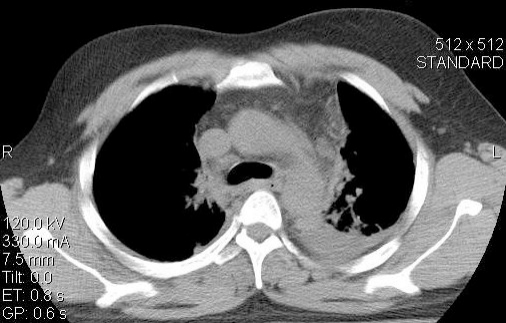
Thorax-CT of the patient indicating left hemothorax, left lung contusion in its posterior segments and a diffusing periaortic hematoma in the aortic isthmus area.
